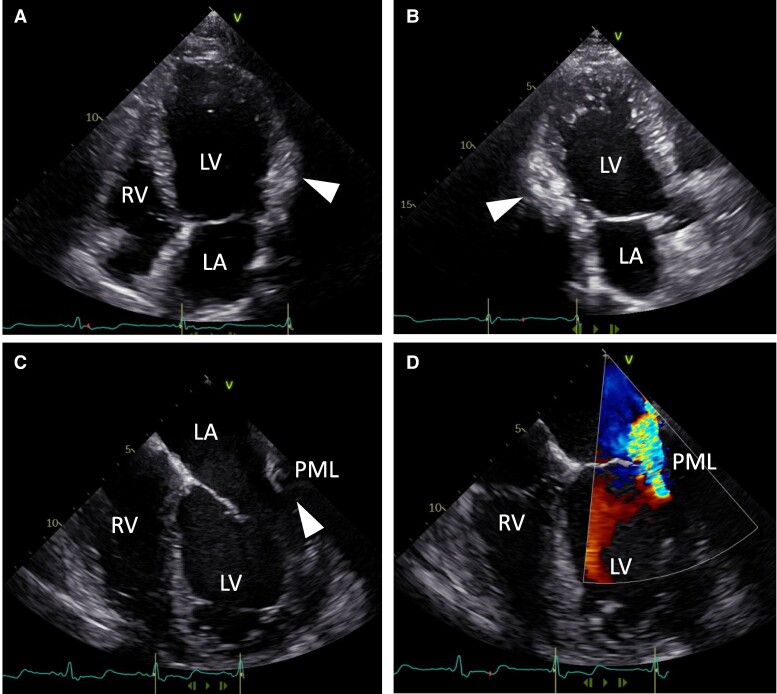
Transthoracic echocardiography showing only the margins of the left ventricular mass (arrowheads) in the apical four-chamber (*A*) and three-chamber (*B*) views. Transoesophageal echocardiography delineates the tethering (arrowhead, *C*) of the mass to the posterior mitral leaflet (PML) causing mitral regurgitation (Doppler jet, *D*). RV, right ventricle; LV, left ventricle; LA, left atrium.
